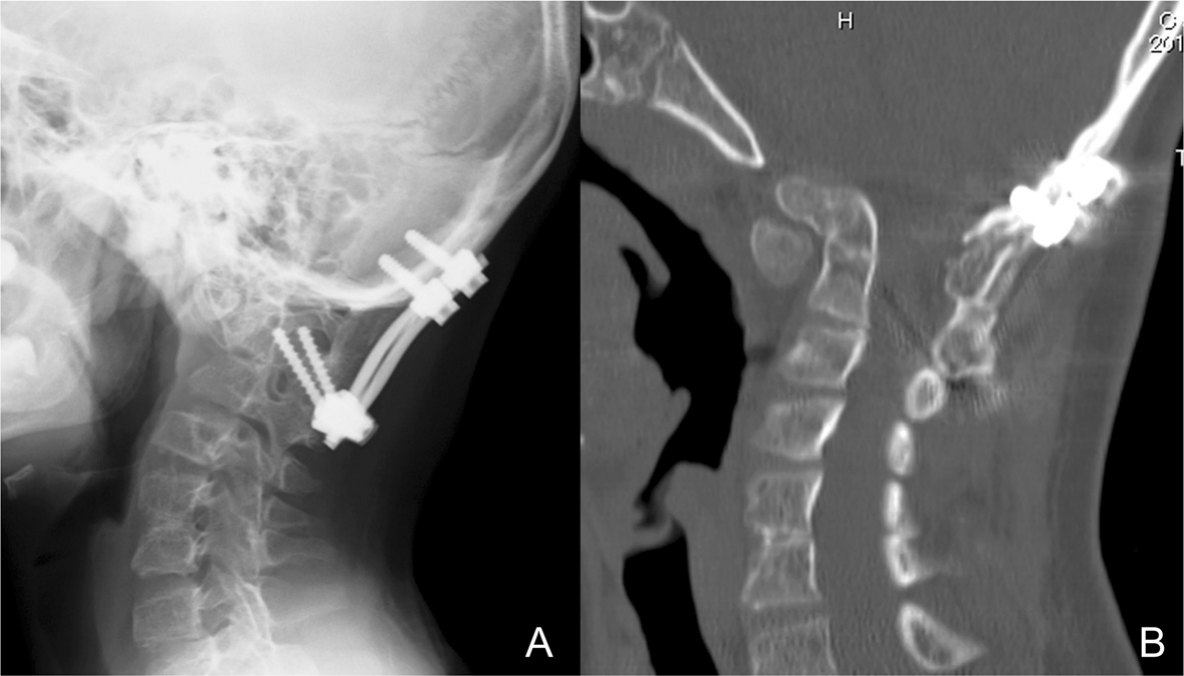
Postoperative imaging of the craniovertebral junction. (A) Lateral radiography shows improved alignment of the craniovertebral junction. (B) A midsagittal reconstruction of computed tomography obtained 4 months postoperatively shows complete bone union.
